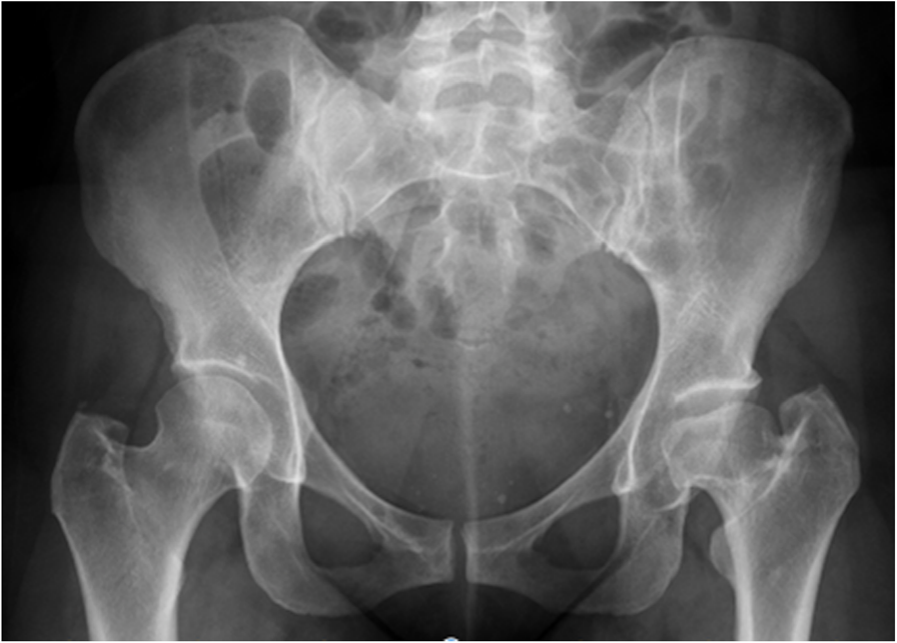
X-ray of a dysplastic left hip with short neck and flattened head
